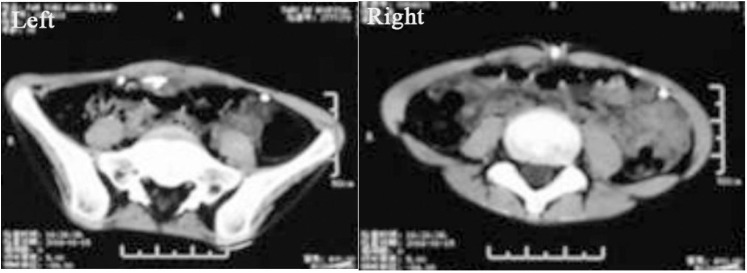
Abdominal axial CT (left) showing that there is fluid around the shunt at the superficial part of the abdomen around the umbilicus. CT (right) demonstrating that the shunt catheter was extruded through the umbilicus.
